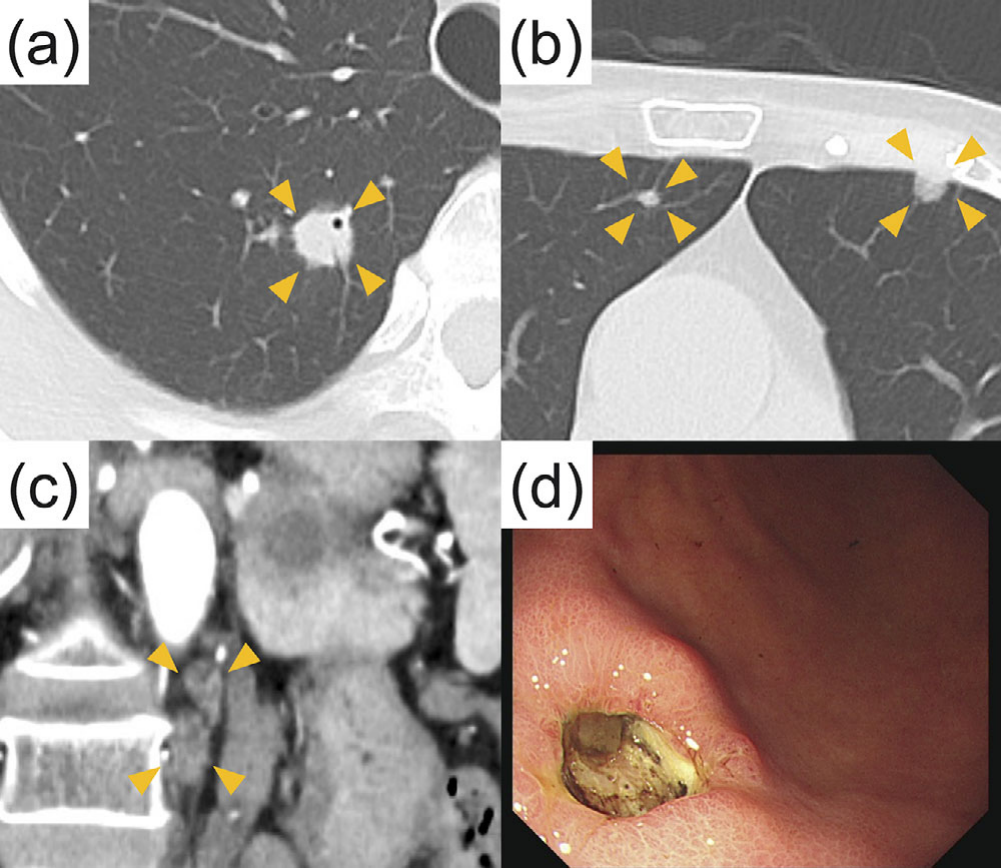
(a, b) Chest computed tomography results: Multiple round masses were found in both lungs (orange arrowheads), raising suspicions of metastatic lung cancer. (c) Abdominal computed tomography results (late arterial phase). Multiple enlarged lymph nodes were found around the aorta (orange arrowheads). (d) Images obtained following upper gastrointestinal endoscopy performed concurrently with endoscopic ultrasound. A solitary ulcer on the anterior wall of the greater curvature in the lower gastric body was detected.
